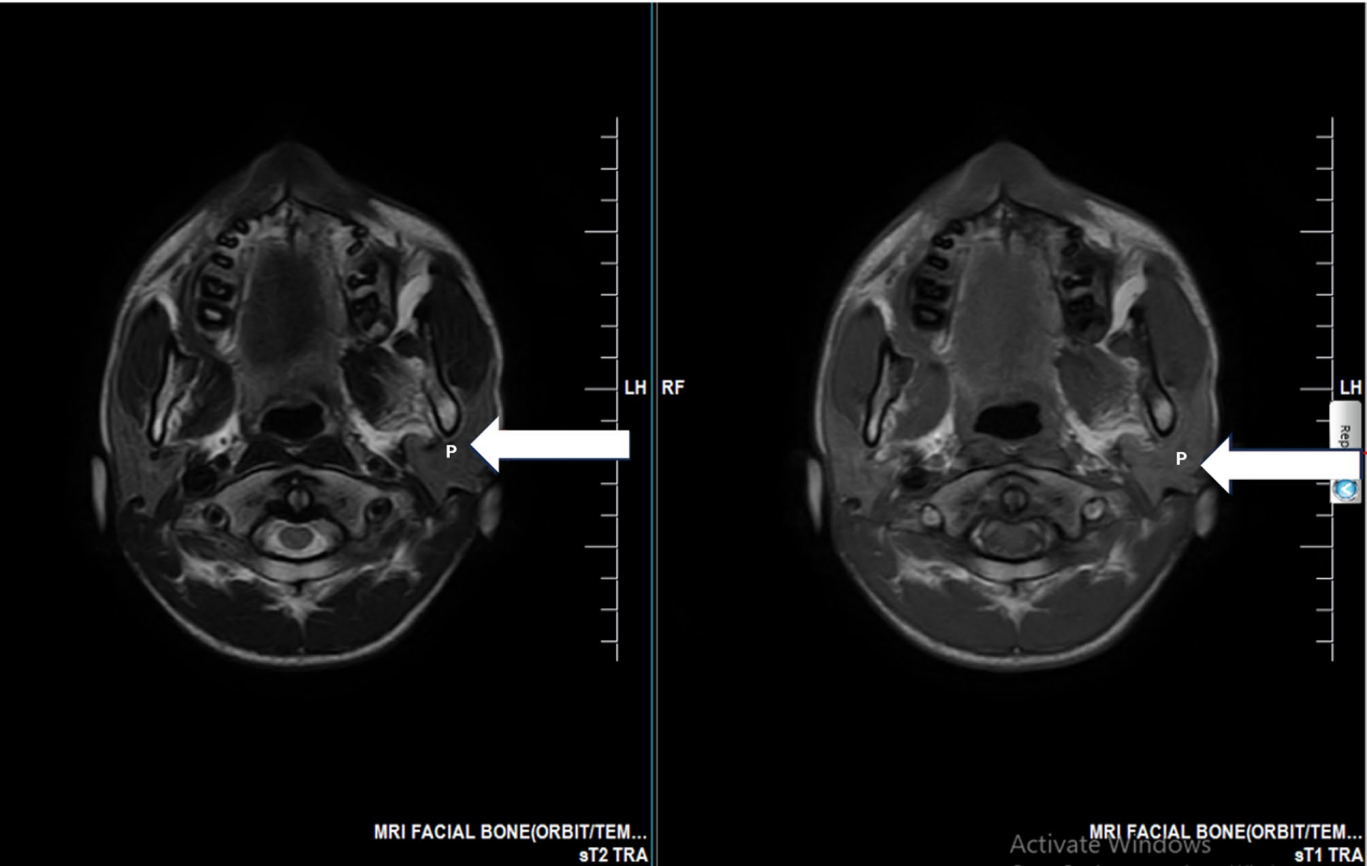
Axial MRI showing unilateral parotid gland enlargement. T2-weighted (left) and T1-weighted (right) axial images demonstrating a bulky and enlarged left parotid gland (white arrowhead, *P* = Parotid) compared to the normal-sized right parotid gland
